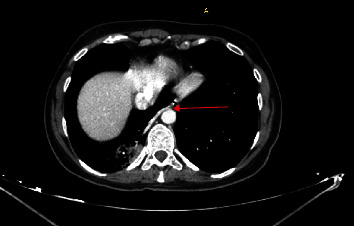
Axial view of the lower chest CT scan angiography showing the solitary aberrant artery (red arrow) originating from the left anterior border of the descending thoracic aorta and running rightward to supply the sequestered segment of the right lower lobe of the lung.
